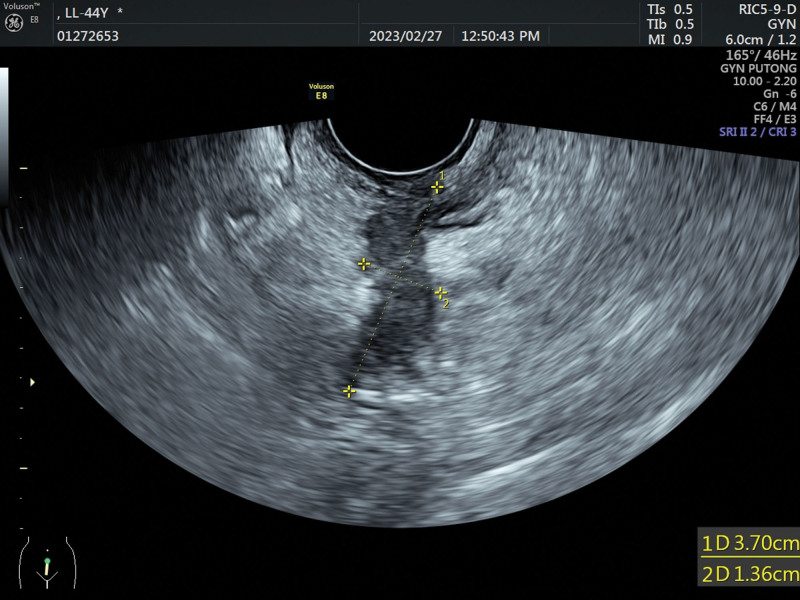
The ultrasound examination suggested a 3.7*1.4 cm hypoechoic cluster with a crab foot distribution to the canal wall.
